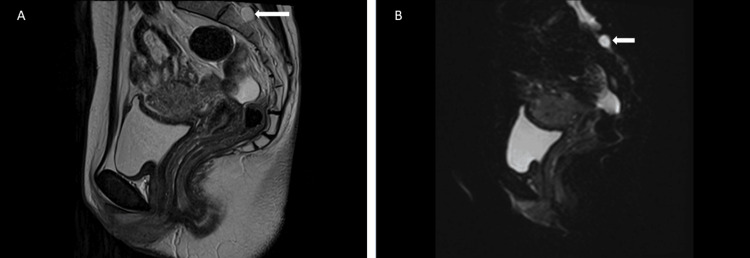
Magnetic resonance imaging of the pelvis Figure 1A: The sagittal T2-weighted image demonstrates a 13mm sacral perineural cyst at the S2 level (white arrow); Figure 1B: The sagittal diffusion-weighted image demonstrates that the signal intensity of the cyst is the same as that of the cerebrospinal fluid (white arrow).
